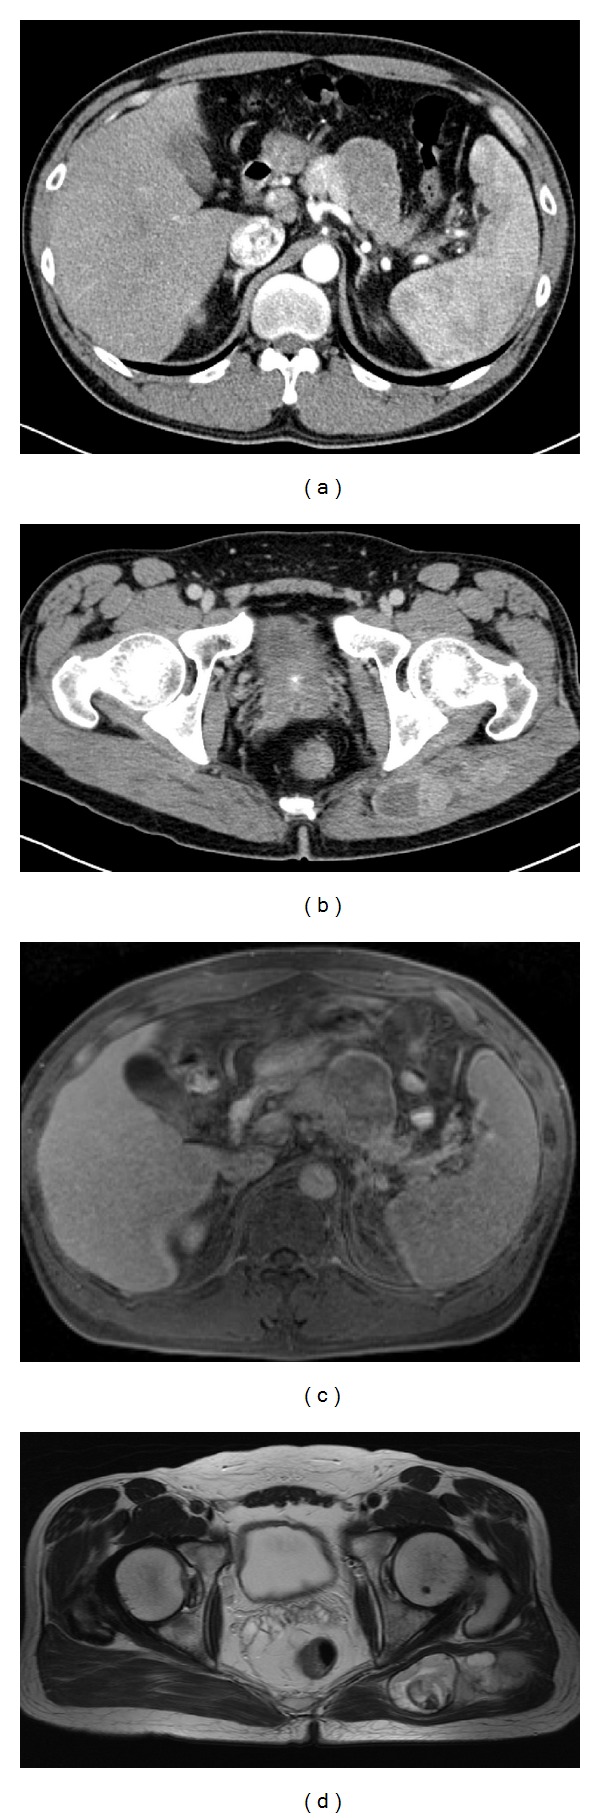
(a) Abdominal computed tomography, showing a well-circumscribed, low-attenuation, inhomogeneously enhancing mass measuring about 5 cm in diameter in the body and tail of the pancreas. (b) Abdominal computed tomography, showing a well-circumscribed, heterogeneously enhancing mass measuring about 8 cm × 3 cm in the left buttock. (c) Abdominal magnetic resonance imaging, showing a well-circumscribed mass measuring 4.5 cm in diameter in the body and tail of the pancreas. The mass had inhomogeneous enhancement with gadolinium diethylenetriaminepentaacetic acid on T1-weighted fat-suppression images. (d) Magnetic resonance imaging of the lesion in the left buttock, showing an inhomogeneously hyperintense mass on T2-weighted images.
